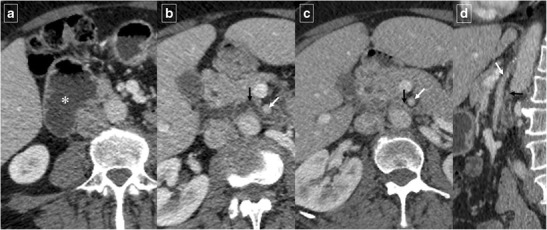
A 31-year-old woman with suspected superior mesenteric artery (SMA) syndrome. Axial contrast-enhanced CT images (a, b, and c) show a dilated proximal duodenum (*) with abrupt narrowing of the third portion (black arrows) corresponding with the course of SMA (white arrow). Reformatted sagittal contrast-enhanced CT image demonstrates compression of the third portion of the duodenum (black arrow) between the SMA (white arrow) and aorta
